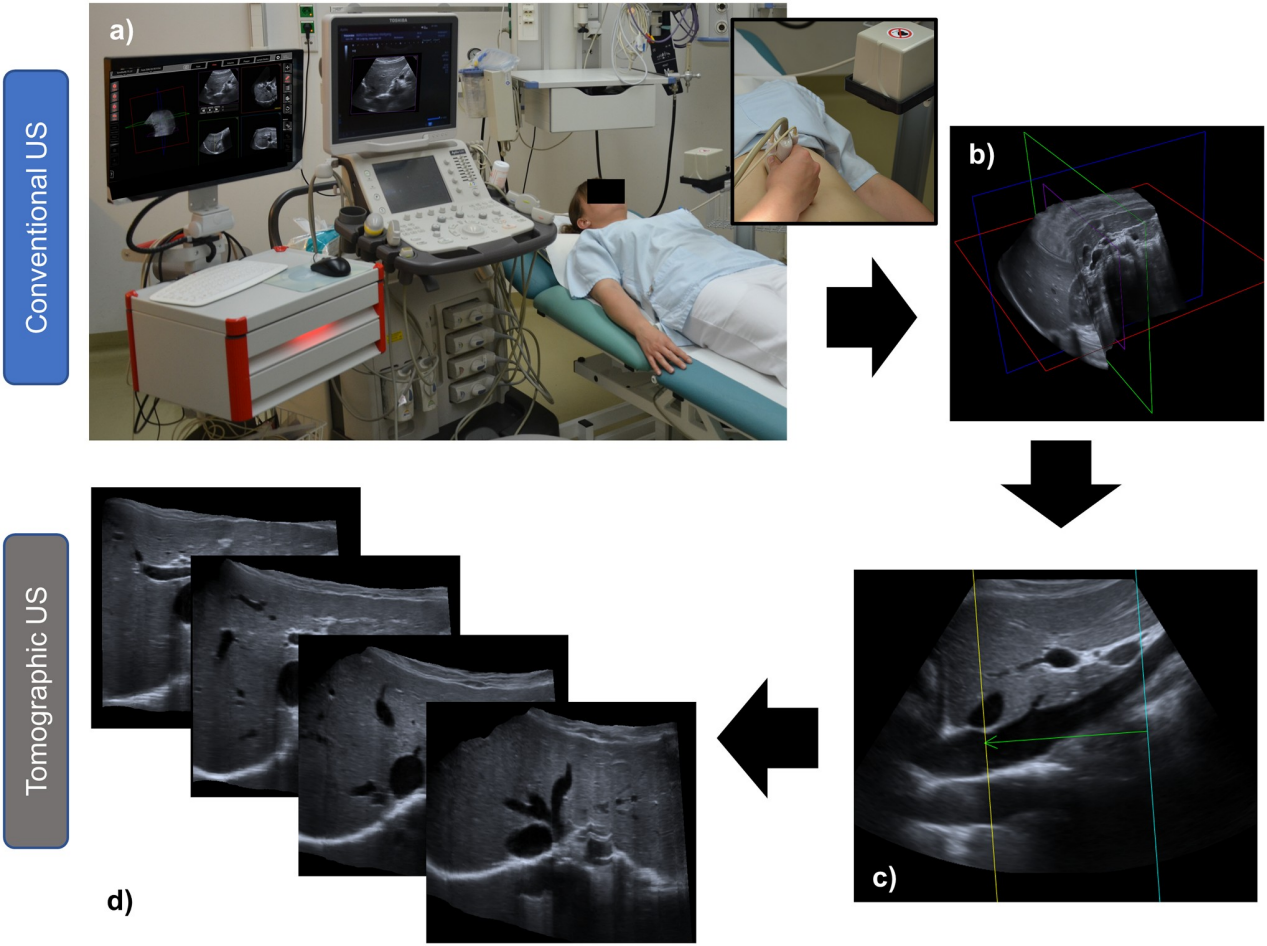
Acquisition of tomographic ultrasound. Conventional ultrasound image-data and spatial information from the tracking system (a) were continuously recorded during a whole organ scan. The tomographic volume (b) was alined in sagittal orientation (c) and series of transversal planes were computed (d). The person (a) gave written permission to use this photo.
